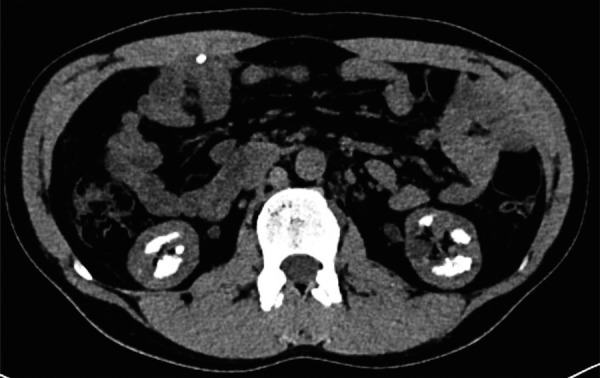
Abdomen CT (transversal view) showing medullary nephrocalcinosis.
